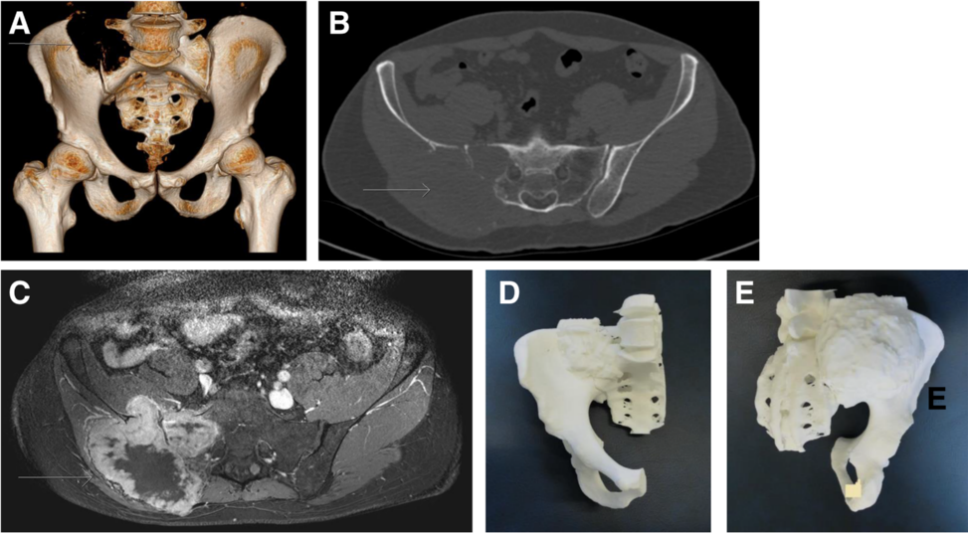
Case 2: preoperative planning. CT (5-A-B) and MRI (5-C) of a giant cell tumor (*arrows*) affecting the iliosacral joint and in close proximity to the first sacral nerve root as well as preoperative planning (5-D-E) with a three-dimensionally printed models of the right hemipelvis including the sacrum and the tumor. a Three-dimensional (3-D) reconstruction of the pelvis. b Axial plane of the CT showing the osteolytic lesion. c T1 contrast-enhanced axial MRI plane. d Anterior view of the 3-D printed model. e Posterior view of the 3-D printed model
